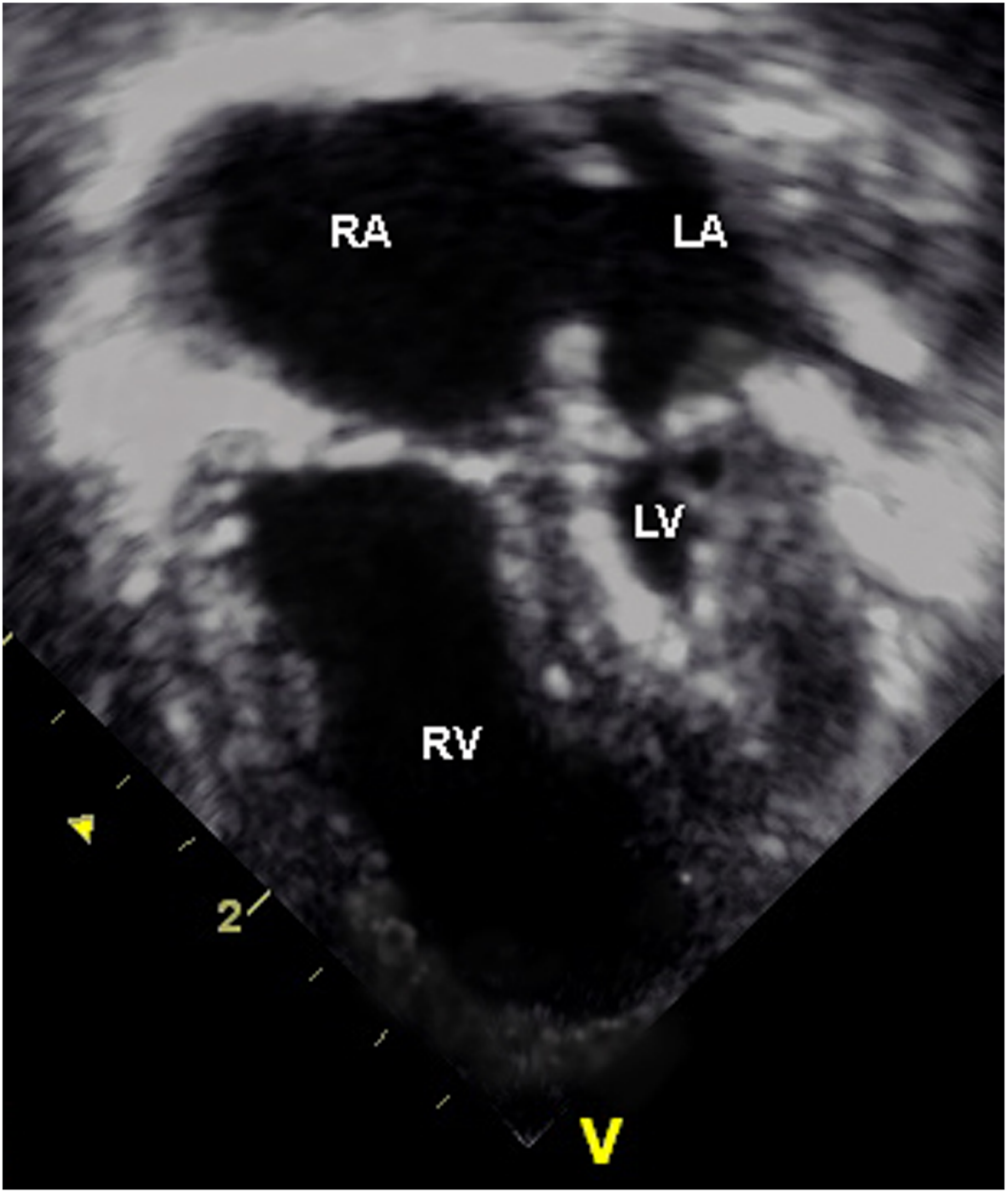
Postnatal transthoracal echocardiography (four chamber view): severe hypoplasia of the left ventricle with endocardial fibroelastosis and hypoplasia of the left atrium are seen. Abbreviations: LA, Left atrium; LV, Left ventricle; RA, Right atrium; RV, Right ventricle.
